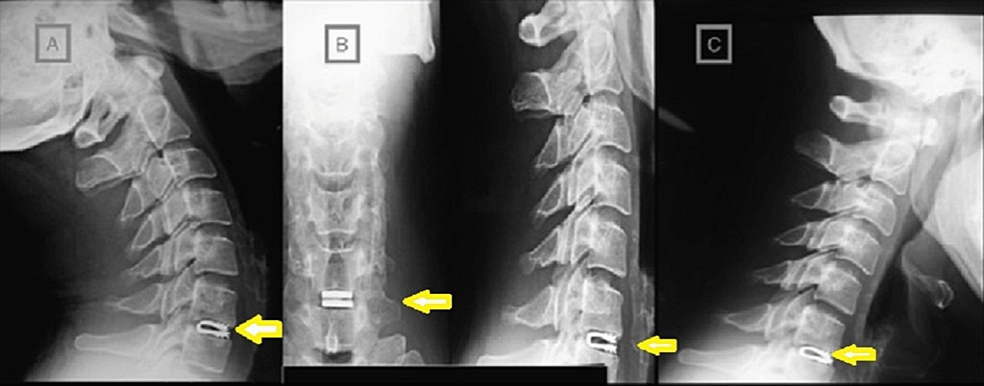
Dynamic (A, C) and plain (B) radiographs of a patient with DCI (arrows) DCI, dynamic cervical implant
